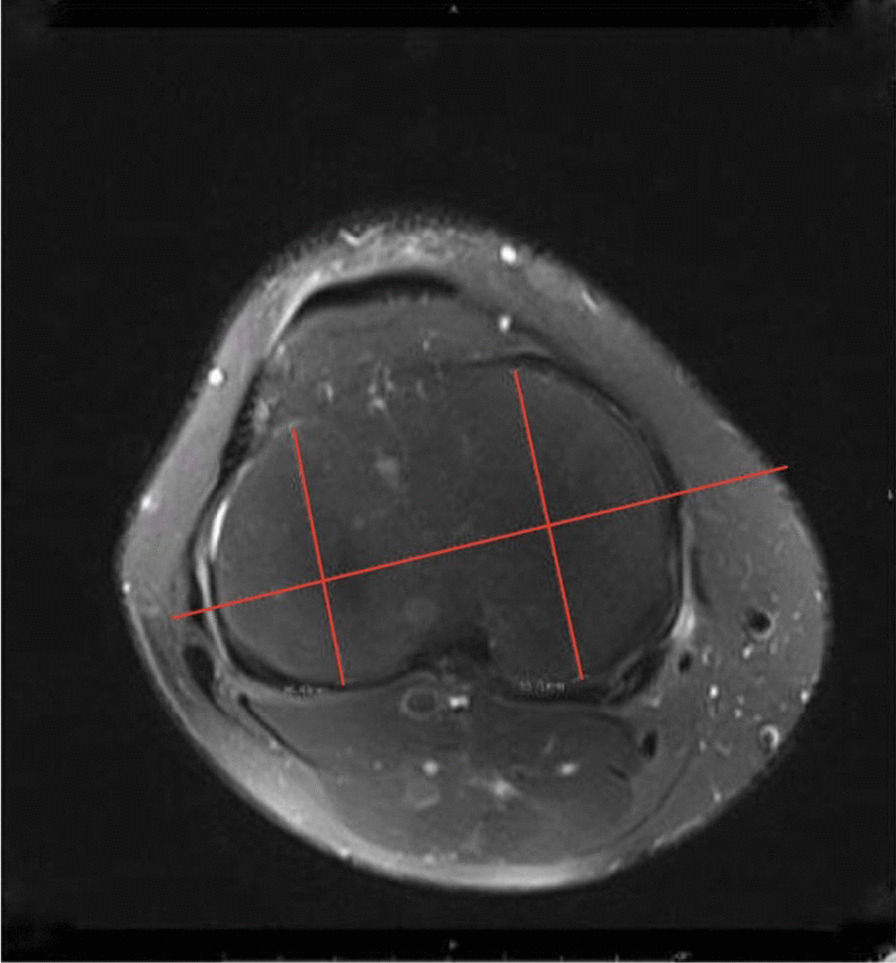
The measurement of medial tibial plateau length on a T2 axial view magnetic resonance image
